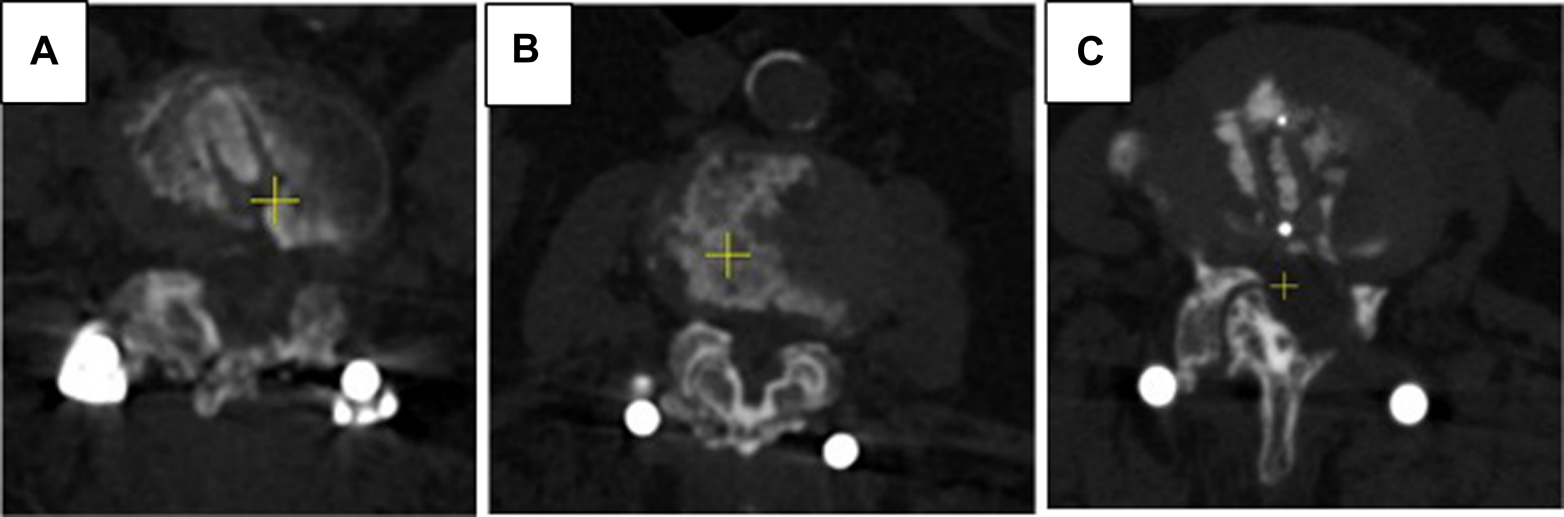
Grades of posterior fusion. (A) CT scans in axial plane, 1 year after surgery - total ankylosing of facet joints was confirmed. (B) CT scans in axial plane, 1 year after surgery - partial ankylosing of facet joints was confirmed. (C) CT scans in axial plane, 1 year after surgery - complete posterior nonunion was detected.
